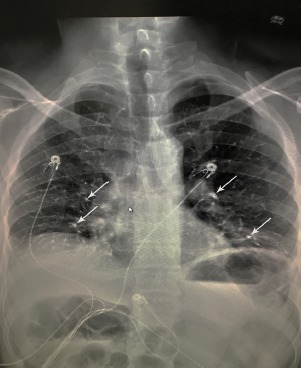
Chest radiograph of a 43-year-old male depicting multiple hyperdense opacities (arrows) with vascular crowding and atelectasis at lung bases.
